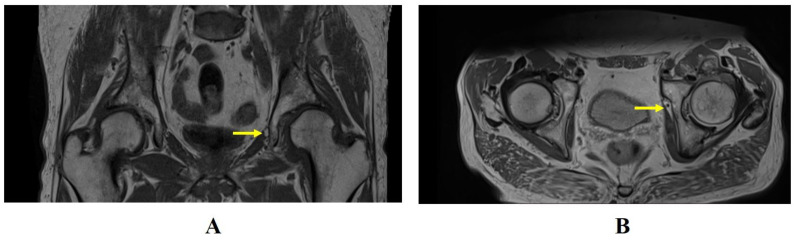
Coronal T1-weighted image (A) and axial PD TSE Dixon image (B). In both images, the right obturator canal is normal, while the left obturator canal demonstrates an abnormal volume of fat accompanying the obturator neurovascular bundle (arrow) as it passes between the obturator muscles. The images are compatible with a type I obturator hernia.
